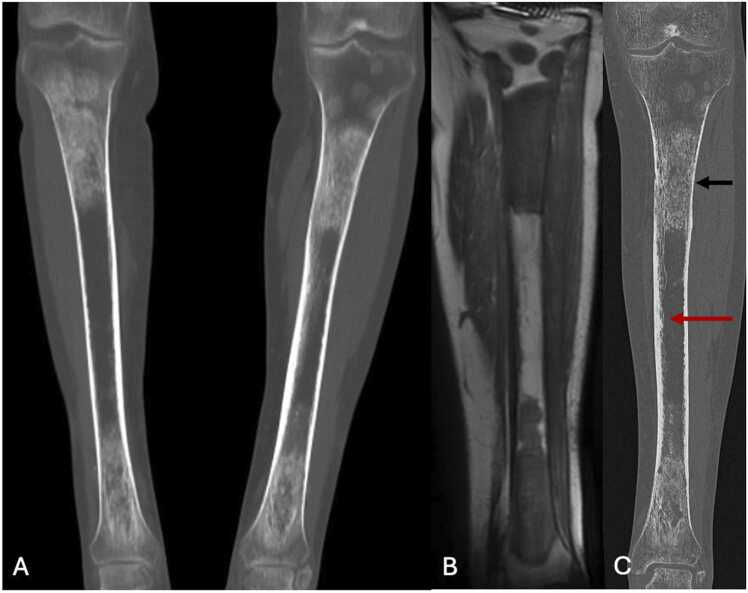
Erdheim-Chester disease in a 50-year-old man. A. Reformation in coronal plane of bone scintigraphy showing bilateral and symetric osteosclerosis of proximal and distal metadiaphyseal junctions of tibias. B. T1-weighted MR image of the left tibia in coronal plane showing marked hyposignal of those sclerotic areas. C. UHR CT of the left tibia in coronal plane highlighting those sclerotic areas of the medulla (black arrow) with better visualization of endosteal involvement of cortical thickening in the middle diaphysis (red arrow).
